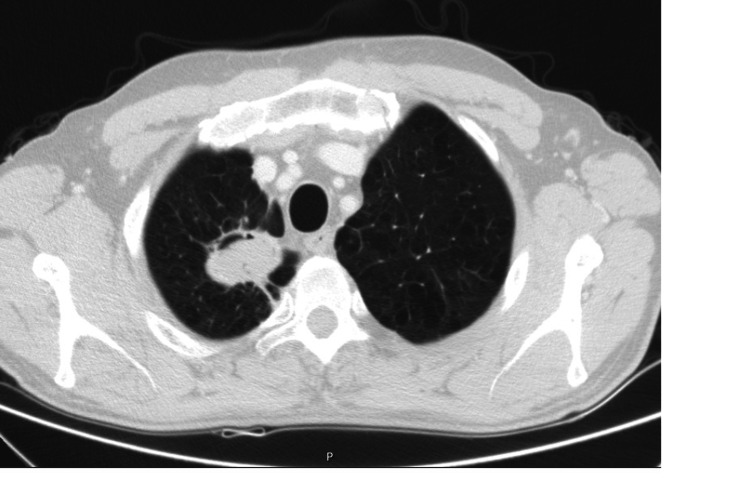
Follow-up abdominal and chest CT after 15 cycles of pembrolizumab in July 2021
